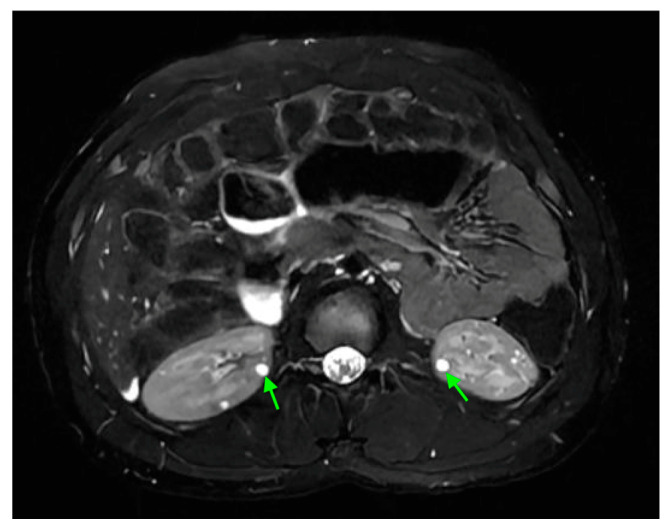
Abdominal MRI of a 16-year-old female with tuberous sclerosis, showing bilateral renal cysts (indicated by green arrows).
